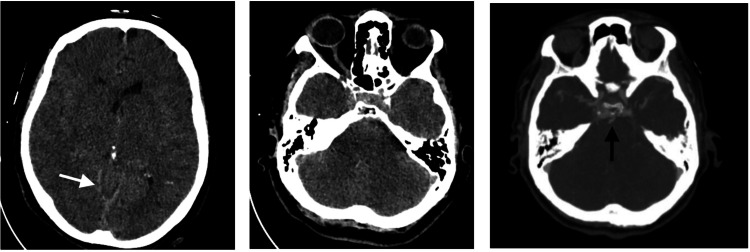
Brain computed tomography of patient 1 There is a diffuse loss of differentiation between gray matter and white matter, both supratentorial and infratentorial, with reduced dimensions of the ventricular system and significant protrusion of the cerebellar tonsils into the region of the foramen magnum. There is also a reduction in amplitude and diffuse and symmetric accentuation of hyperdensity in the cisternal spaces as well as the cerebral falx and cerebellar tentorium, with the appearance of "pseudo-subarachnoid hemorrhage" (white arrow). All these aspects suggest the presence of diffuse cerebral edema. Angiography study shows engorgement of venous structures (black arrow), with prominence of the silhouettes of the cavernous sinuses and venous plexus in the craniocervical and upper cervical transition.
